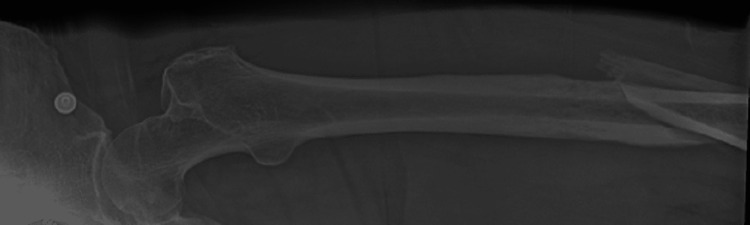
Femur x-ray showing the distal displaced femoral fracture (view 2)
